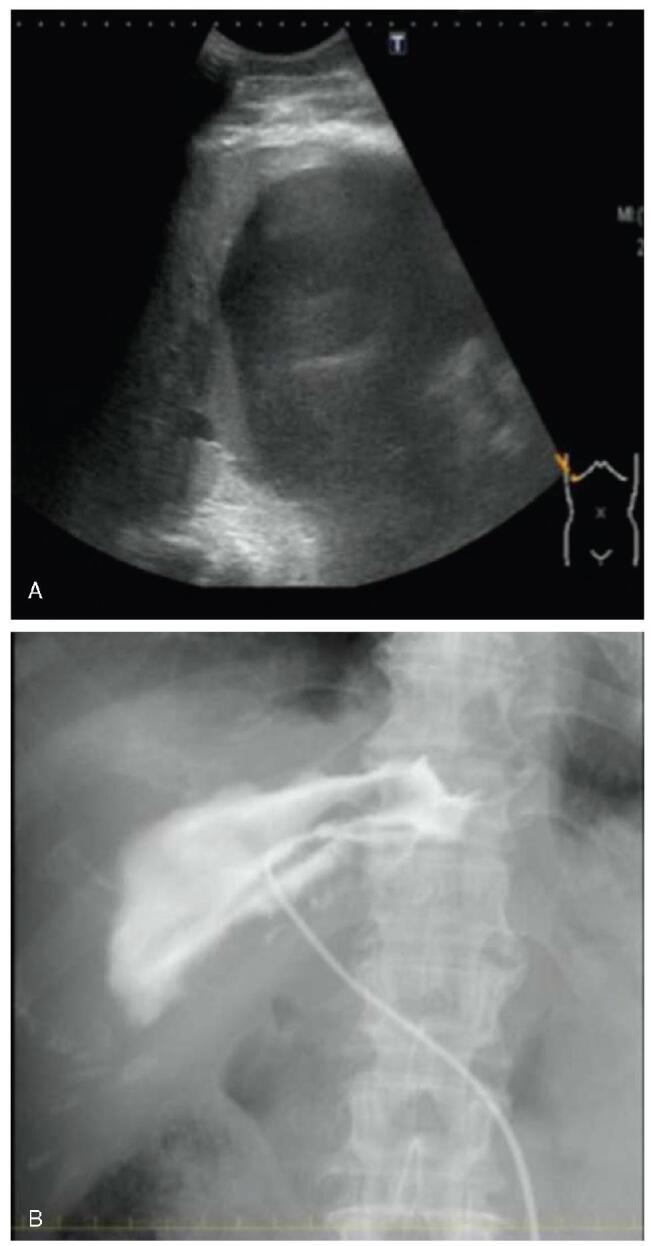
Figure 2. Ultrasonography-guided percutaneous transhepatic cyst drainage was performed for the giant liver cyst. A) Ultrasound showing the liver cyst. B) Fluoroscopic image showing the stent placed in the cyst.
